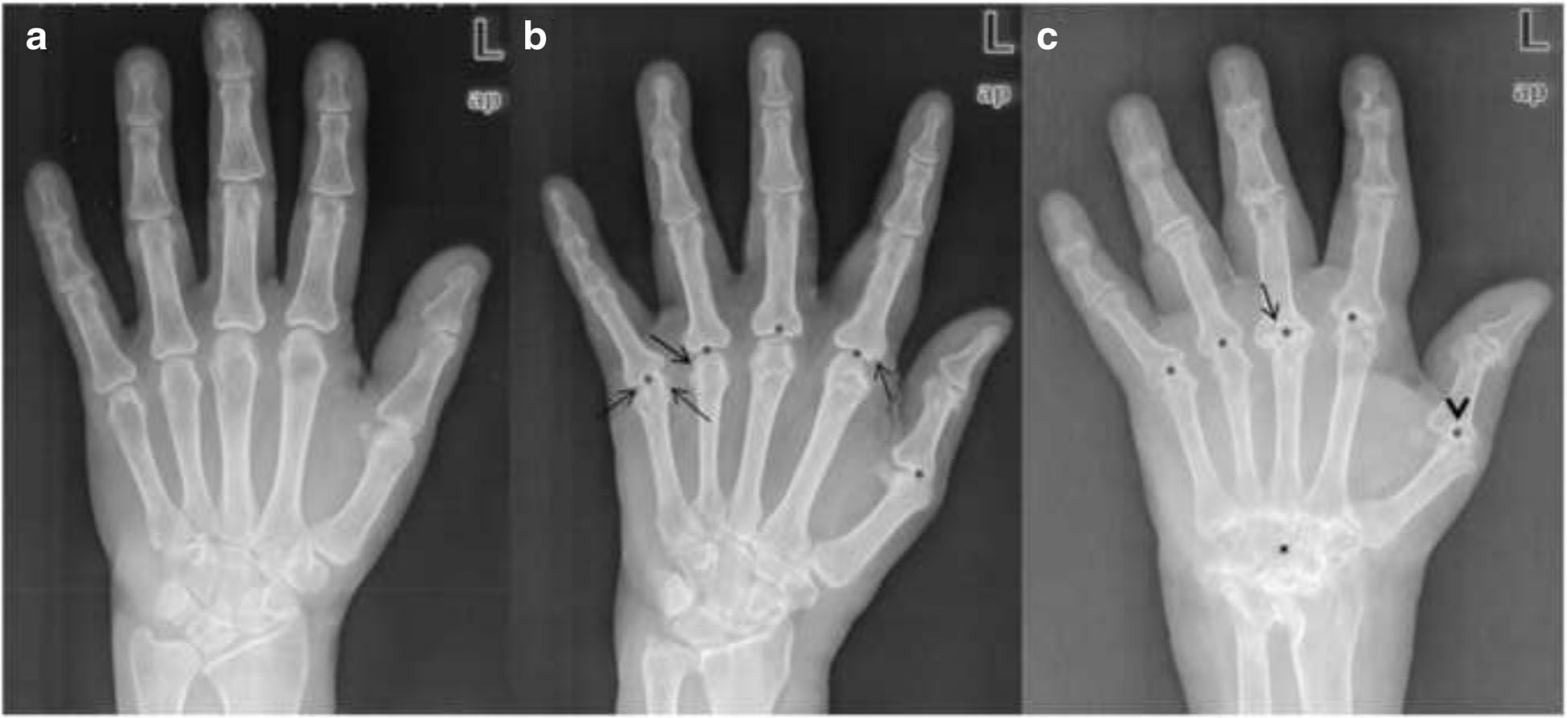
a Normal hand X-ray without signs of RA b Demineralisation of the metacarpal bone, joints space narrowing of the metacarpophalangeal joint I to V (*asterix*) and erosions of the metacarpophalangeal joint (*arrows*) c Severe demineralisation of the metacarpal bones as well as advanced joint space narrowing of the carpus and metacarpophalangeal joint I to V (*asterix*) with total loss of joint space at the metacarpophalangeal joint II and III, erosive destruction of the metacarpophalangeal joint III and subluxation of the metacarpophalangeal joint I
